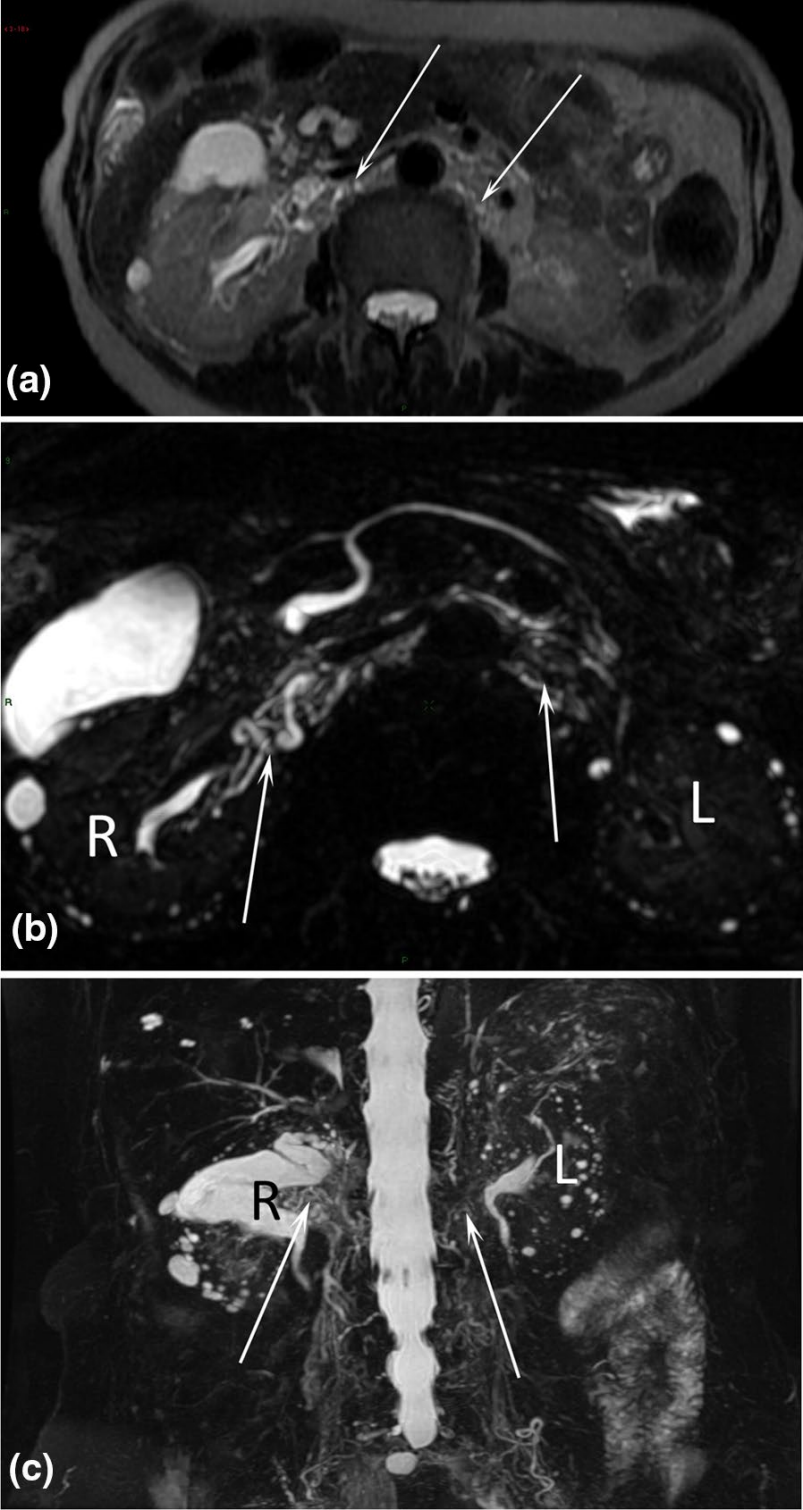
83-year-old female patient with chyluria secondary to filariasis. Axial T2-weighted MR image a demonstrated dilated and enlarged lymphatic vessels prevailing on the right side in contact with the right and left kidneys (arrows). The dilated lymphatic vessels (arrows) were better highlighted on MR lymphography with MIP reconstruction in the axial (b) and coronal (c) planes. *R* right kidney, *L* left kidney
